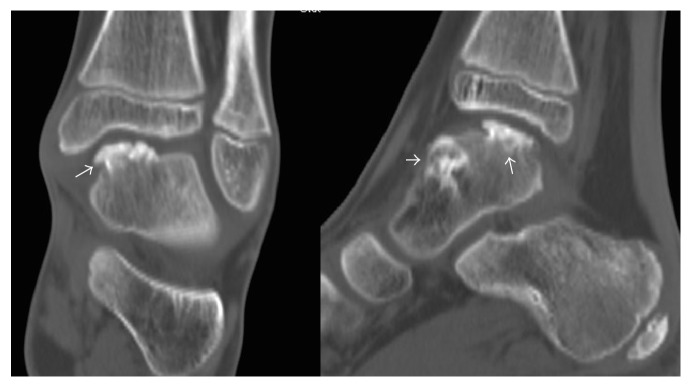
The CT scan shows the bony-like protuberances arising at the anteromedial and posteromedial aspect of the talus (arrows) and the relationships with the other bone structures of the ankle.
